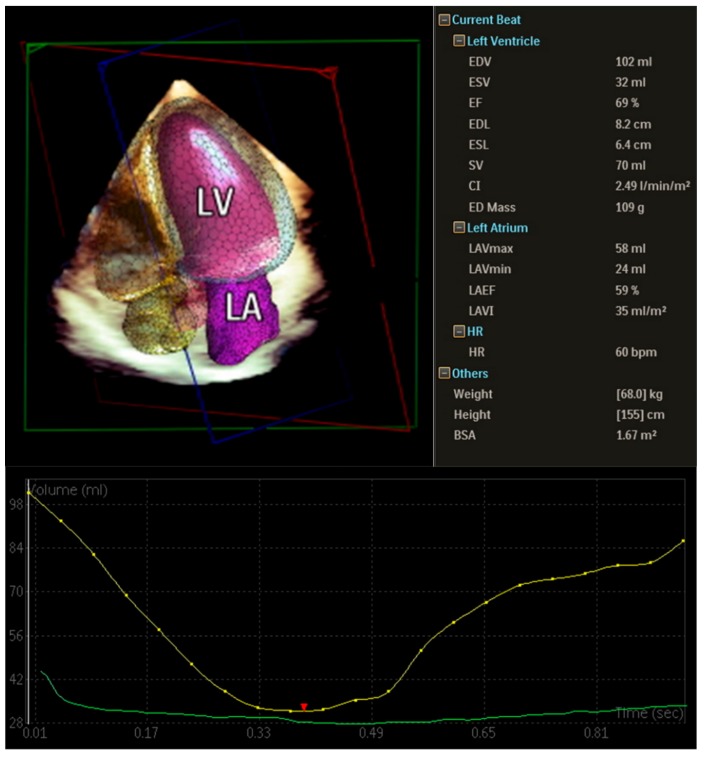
3D echocardiography. Semi-automatic calculation of cardiac chamber dimensions, systolic function, atrial function, and left ventricular mass.
